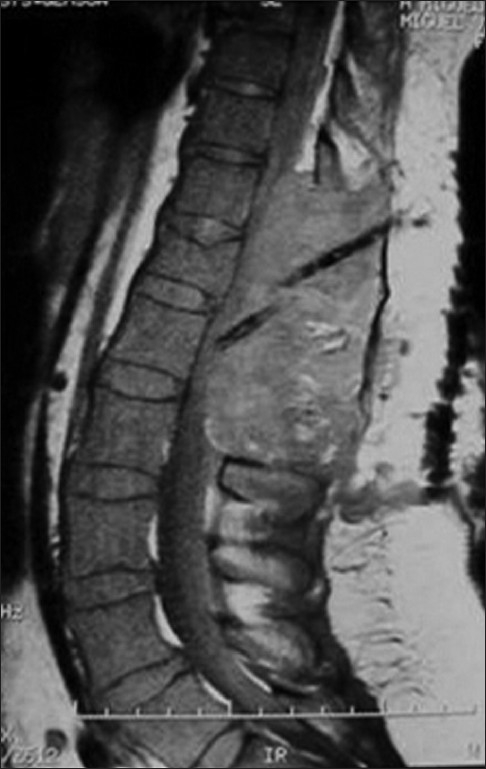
Postoperative saggital MRI of the thoracolumbar region (on the third day) showing massive collection of blood
